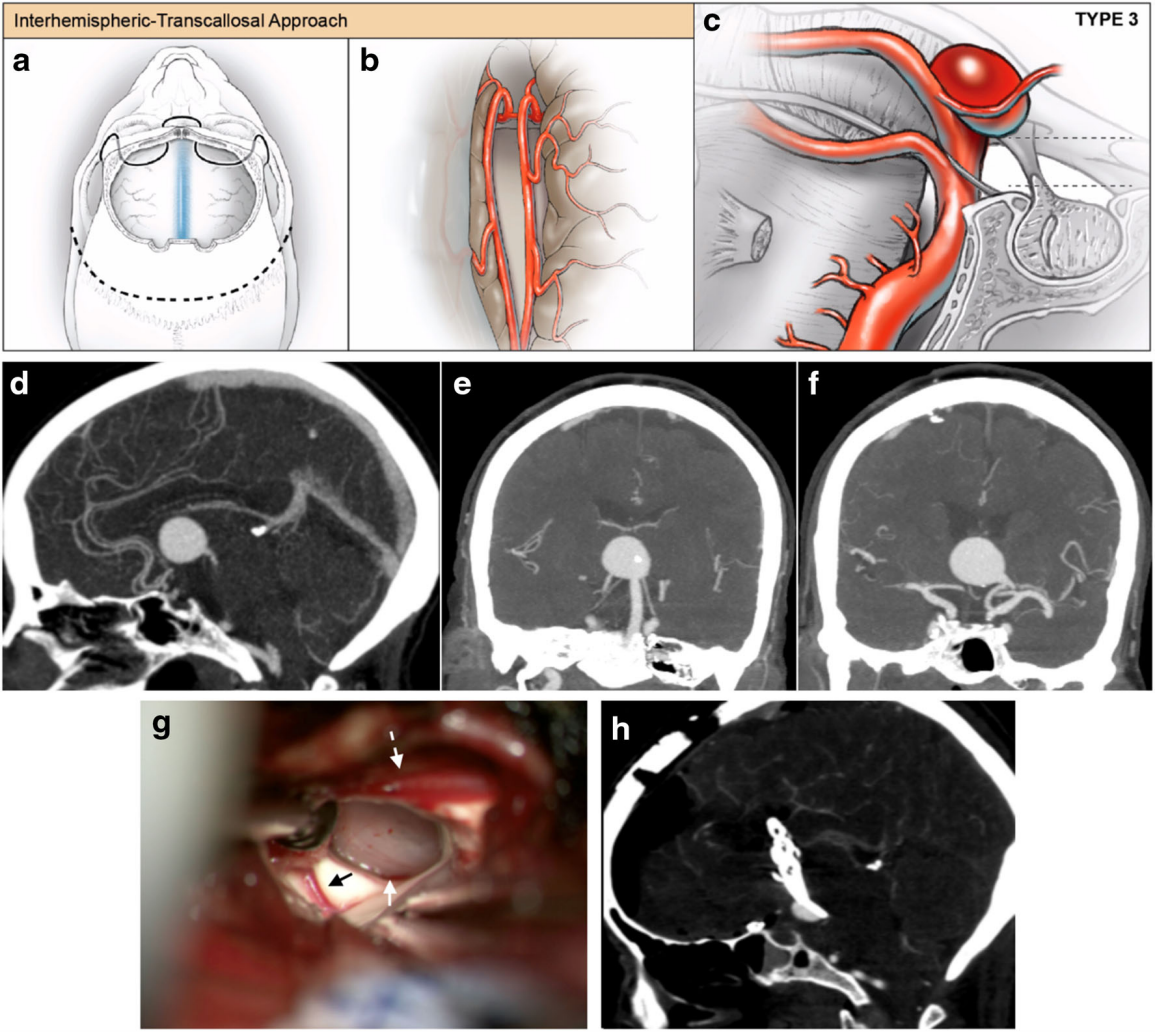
Representative interhemispheric-transcallosal approach to basilar artery aneurysms. (a–c) For basilar apex aneurysms that are significantly superior to the dorsum sella and are projecting into or located completely within the third ventricle, an interhemispheric-transcallosal approach offers visualization of the aneurysm neck. This was performed for one patient in this case series. (a) After a standard bicoronal incision, a bifrontal craniotomy is performed. (b) Interhemispheric dissection is performed to dissect the pericallosal arteries away from the corpus callosum, and the corpus callosum is opened and the right lateral ventricle is entered first. To widen the surgical corridor into the third ventricle, the opening into the third ventricle through the foramen of Monroe is further extended posteriorly by performing a trans-choroidal fissure approach. Simultaneously, a pterional approach was performed for proximal control of the basilar artery trunk via a trans-Sylvian corridor which allowed temporary clipping of the basilar artery and aneurysm softening. (d–h) Representative images of management of an unruptured giant partially fusiform basilar apex aneurysm. (d–f) Preoperative sagittal (d) and coronal (e, f) CT angiogram demonstrates anterior and superiorly projecting aneurysm into the third ventricle. (e, f) Coronal images demonstrate the fusiform nature of the aneurysm with both PCAs originating from the aneurysm dome. (g) Intraoperative photograph demonstrates view through the Foramen of Monroe with aneurysm dome visible (white arrow). The thalamostriate vein (black arrow) and anterior septal vein (dashed white arrow) are visible. The aneurysm is dissected free from ependymal attachments to visualize the neck and clip the aneurysm. (h) Immediate postoperative sagittal CT angiogram demonstrates a base remnant of the fusiform aneurysm from which the posterior cerebral arteries exit
